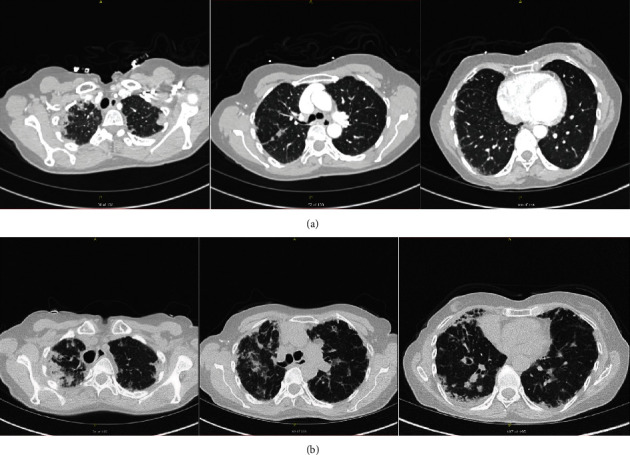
High-resolution computed tomography scans in a patient diagnosed with pleuroparenchymal fibroelastosis (Case 2). (a) Scans in 2015 showed interstitial changes bilaterally, most pronounced in the lung apices, with soft tissue thickening along the major fissure of the right lung between the middle and upper lobes and mild consolidation of the right lung apex. (b) Scans in 2020 showed progression of fibrosis.
